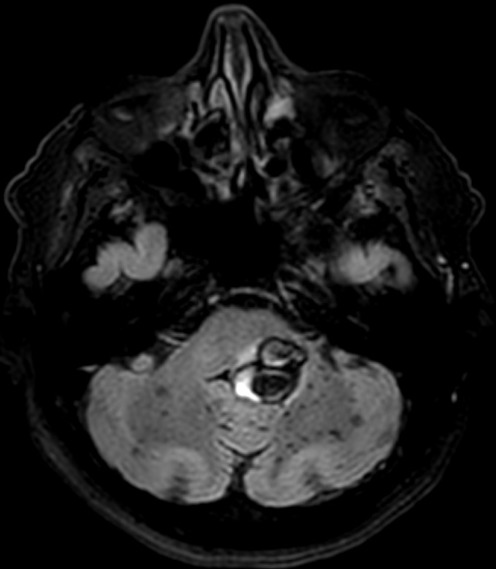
IRM cérébrale, coupe axiale à l’étage infra tentoriel, séquence T2 FLAIR montrant des cavernomes multiples en hyposignal hétérogène « en poivre et sel »
